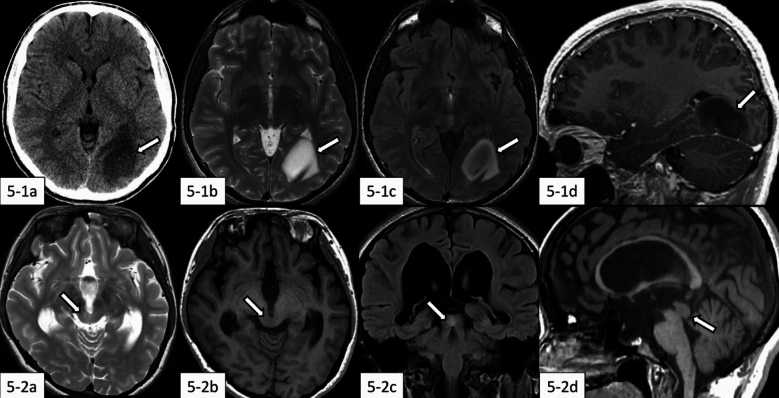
Diffuse low-grade glioma, MAPK pathway-altered. 5-1 (15-year-old boy): There is a tumor mass in the left occipital lobe (5-1a–d, arrows). The tumor mass shows hypointensity on non-contrast computed tomography (5-1a), hyperintensity on T2-weighted imaging (5-1b), peripheral hyperintensity on fluid-attenuated inversion recovery (FLAIR) imaging (5-1c) with positive T2-FLAIR mismatch sign, and lack of contrast enhancement on contrast-enhanced T1-weighted sagittal imaging (5-1d). The same case with different images has been evaluated in our previous study [2]. 5-2 (20-year-old woman): There is a tumor mass in the tectum of the midbrain, accompanied by hydrocephalus (5-2a–d, arrows). The tumor mass shows hyperintensity on T2-weighted imaging (5-2a) and FLAIR coronal imaging (5-2c), and hypointensity on T1-weighted axial and sagittal imaging (5-2b and 5-2d, respectively) with ill-defined margin
